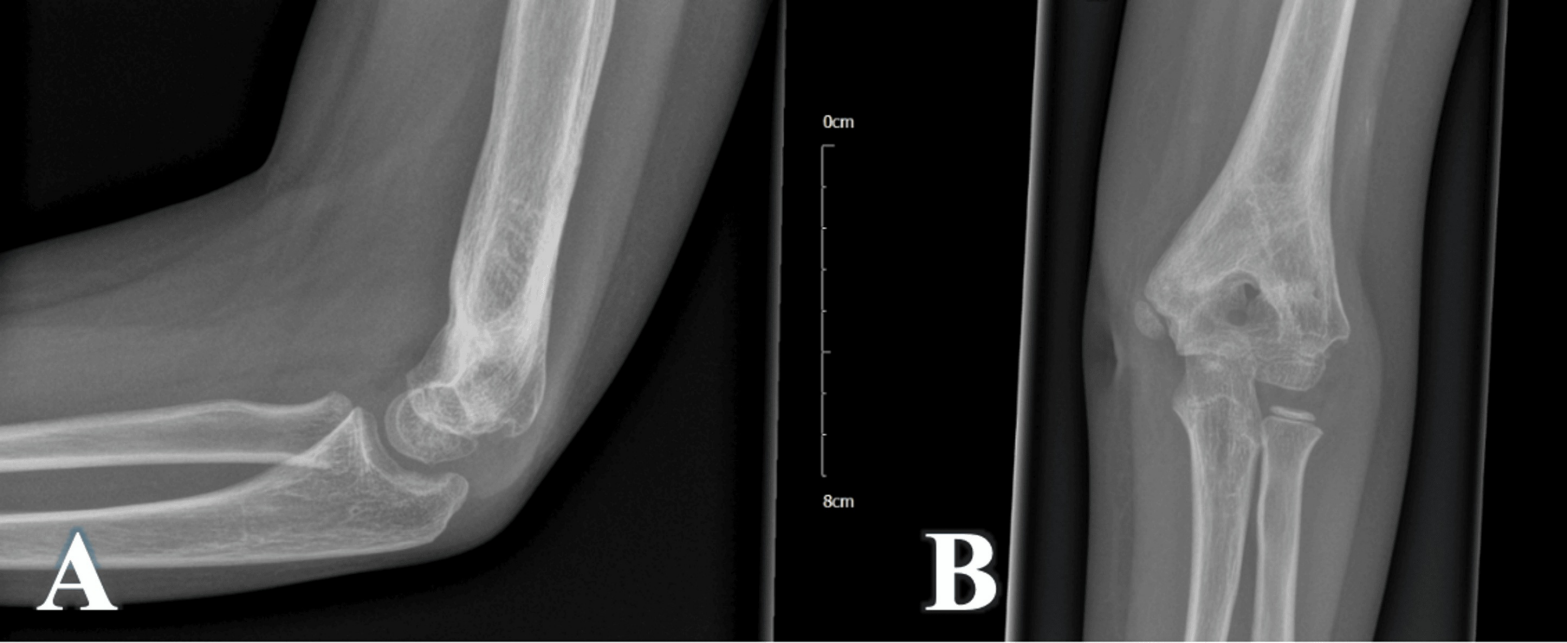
Final follow-up radiographs of the elbow, showing complete resolution A - Lateral view of the elbow; B - Anteroposterior view of the elbow
